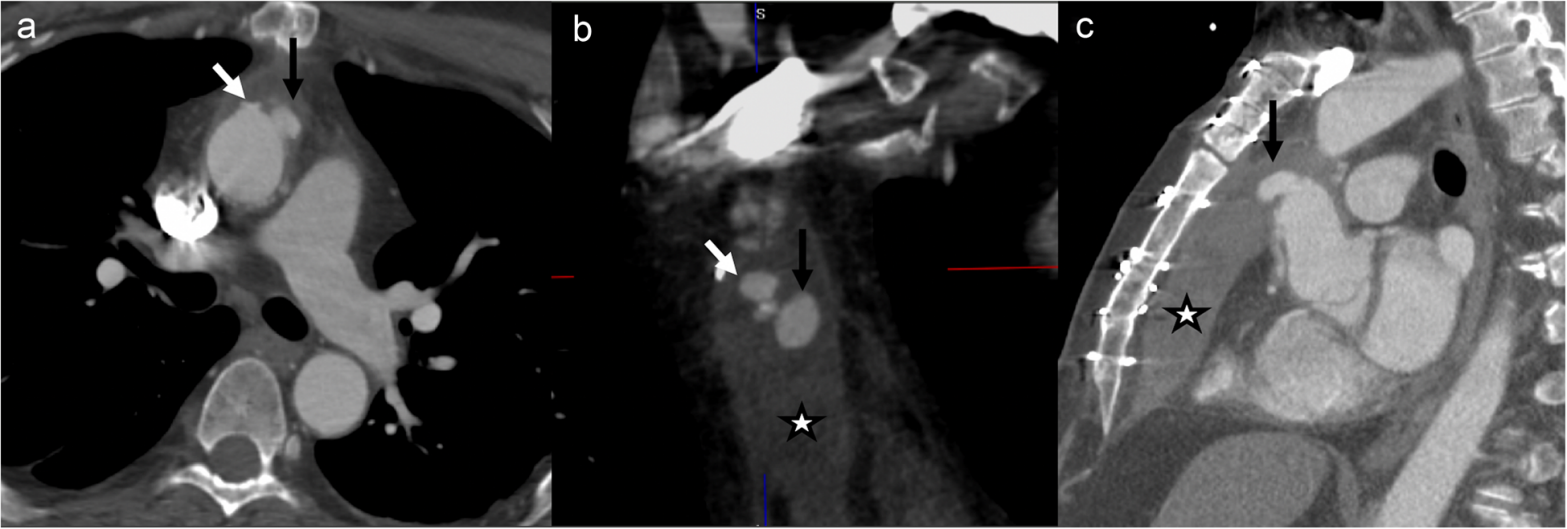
A 69-year-old male, 6-week status post open ascending aortic aneurysm repair with chest pain, presented with acute chest pain. Axial (a), coronal (b), and sagittal (c) CTA images show a smooth outpouching (black arrow) near graft repair consistent with perfusion catheter stump (expected post-surgical finding). Additionally, there is another irregular outpouching (white arrow), and irregularity and associated anterior pericardial hematoma (star) is concerning for a pseudoaneurysm. The patient had an emergent repeat surgical graft repair
